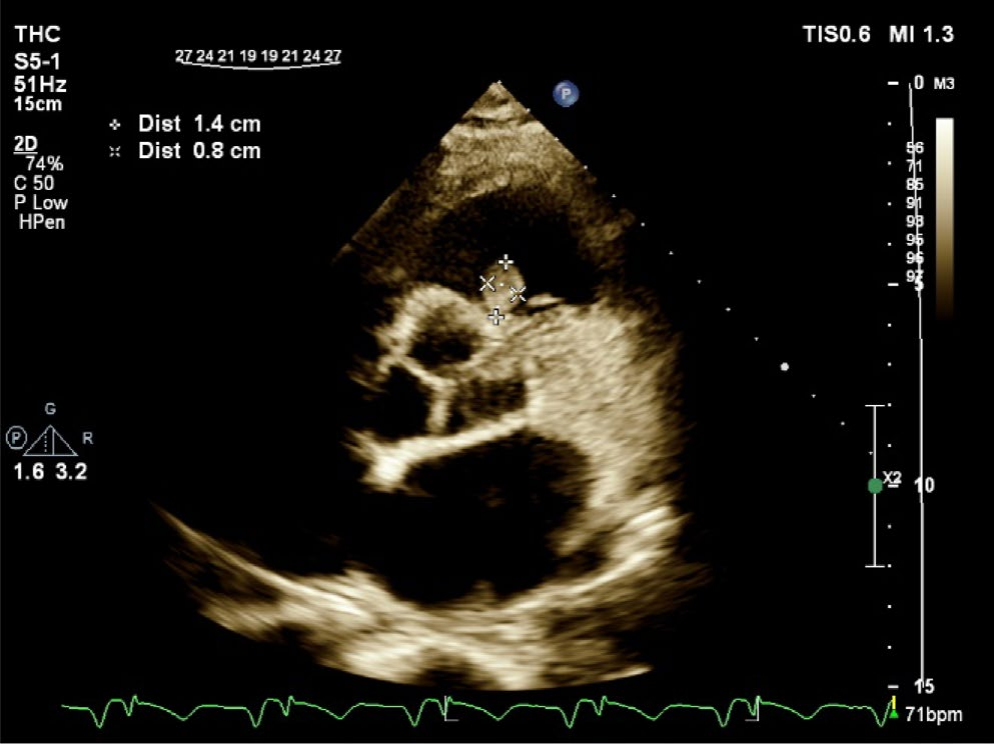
Transthoracic echocardiography in parasternal short axis, showing RVOT mass inserted close to pulmonary valve. RVOT, right ventricular outlet tract.
